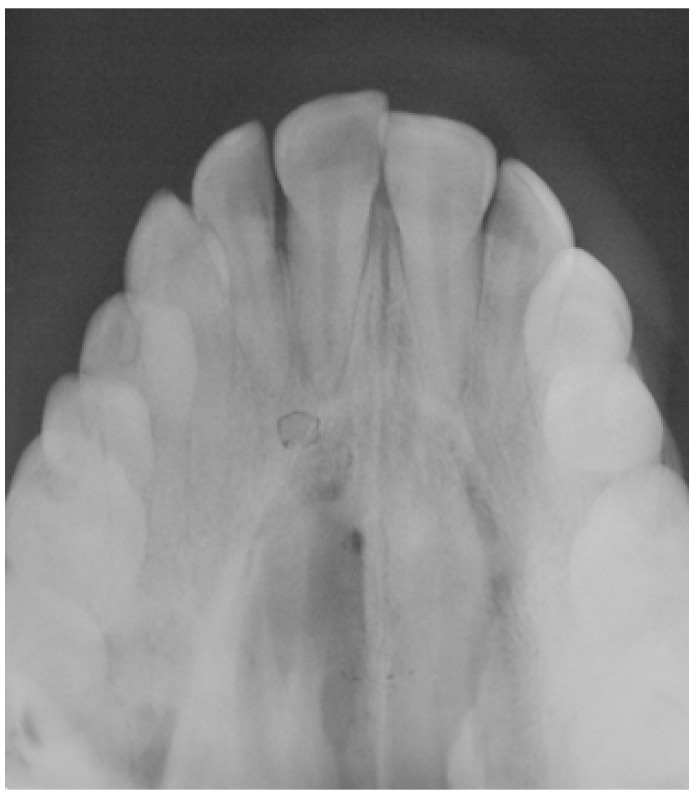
Occlusal radiography showing a not well-defined and slightly more radiolucent area.
